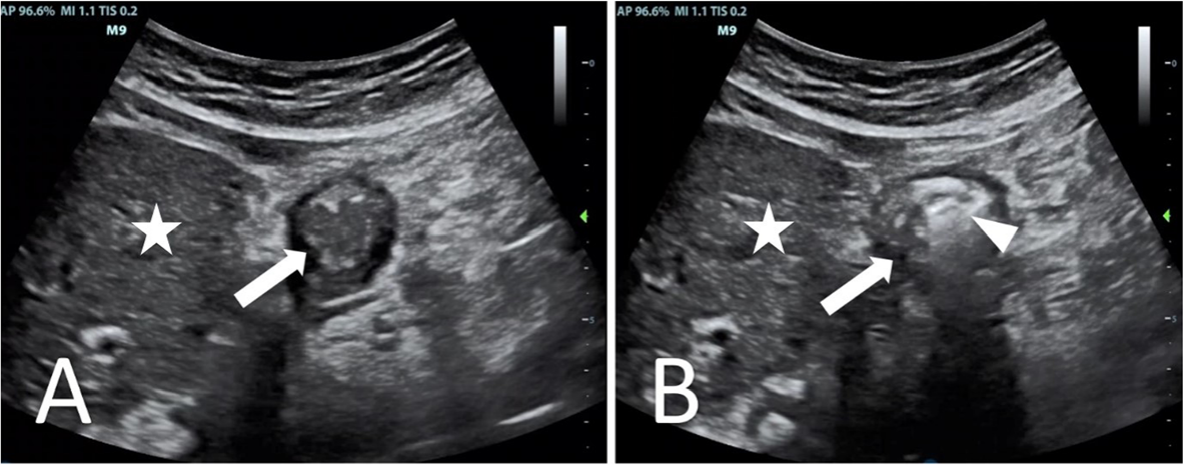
Male, 52 years old, with the chief complain “cough for 1 week and chest distress for 2 days.” A gastric tube was put. a Ultrasound showed the stomach (white arrow) and the liver (white star). b And then 20 ml of air was injected through the NGT; ultrasound showed dynamic hyperechogenic air shadow in the stomach (white triangle)
